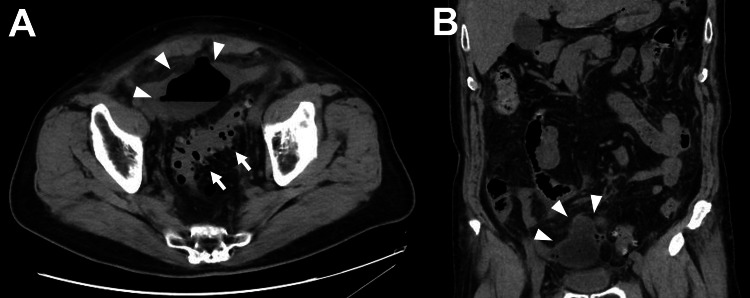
Abdominal computed tomography (A) Axial computed tomography (CT) showing several diverticula at the sigmoid colon (arrow), with inflammation, surrounded by free air, and ascites (arrowheads). (B) Coronal CT showing severe inflammation in the lower abdominal abscess (arrowheads)
